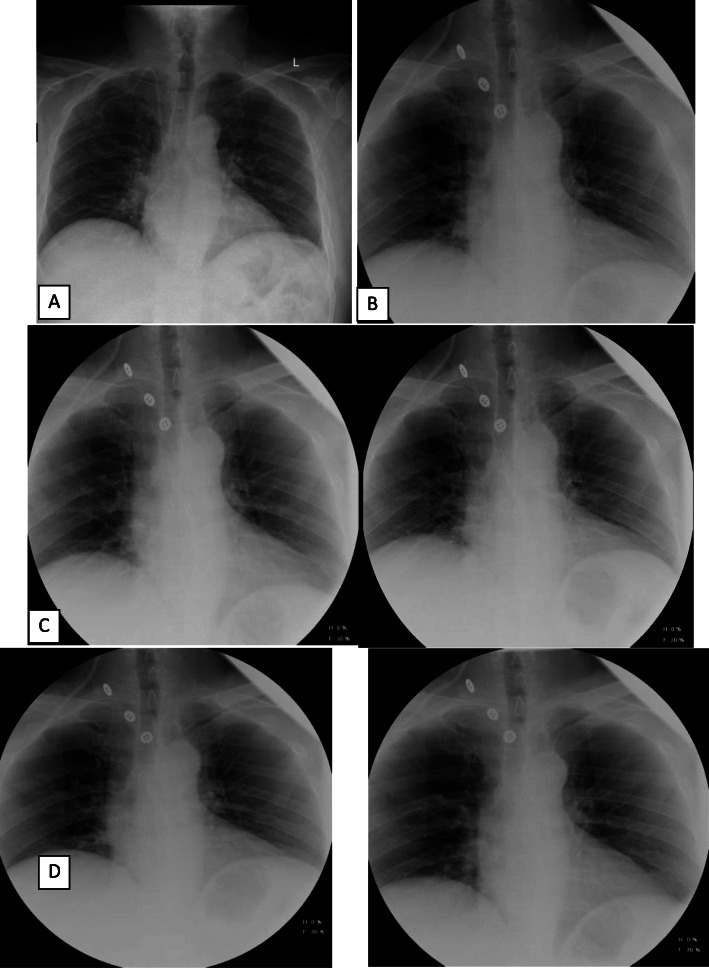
Post-operative postero-anterior chest sniff test x-rays. Posteroanterior chest X-rays, a Preoperative posteroanterior radiograph showing normal height of both hemidiaphragms. b Postoperative posteroanterior radiograph showing normal height of both hemidiaphragms. c On quiet breathing, normal orthograde motion of both hemidiaphragms. d On deep respiration, normal orthograde motion of both hemidiaphragms. No delayed or paradoxical movement
